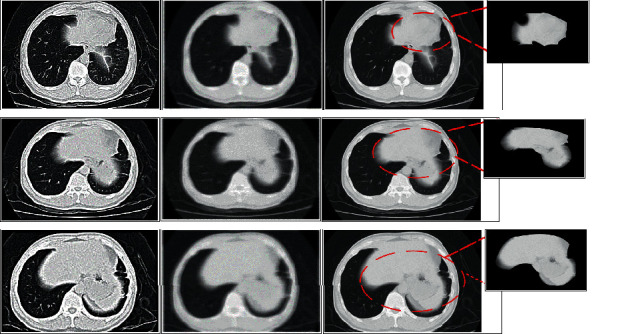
Reconstruction of CT images of lungs by backprojection.
